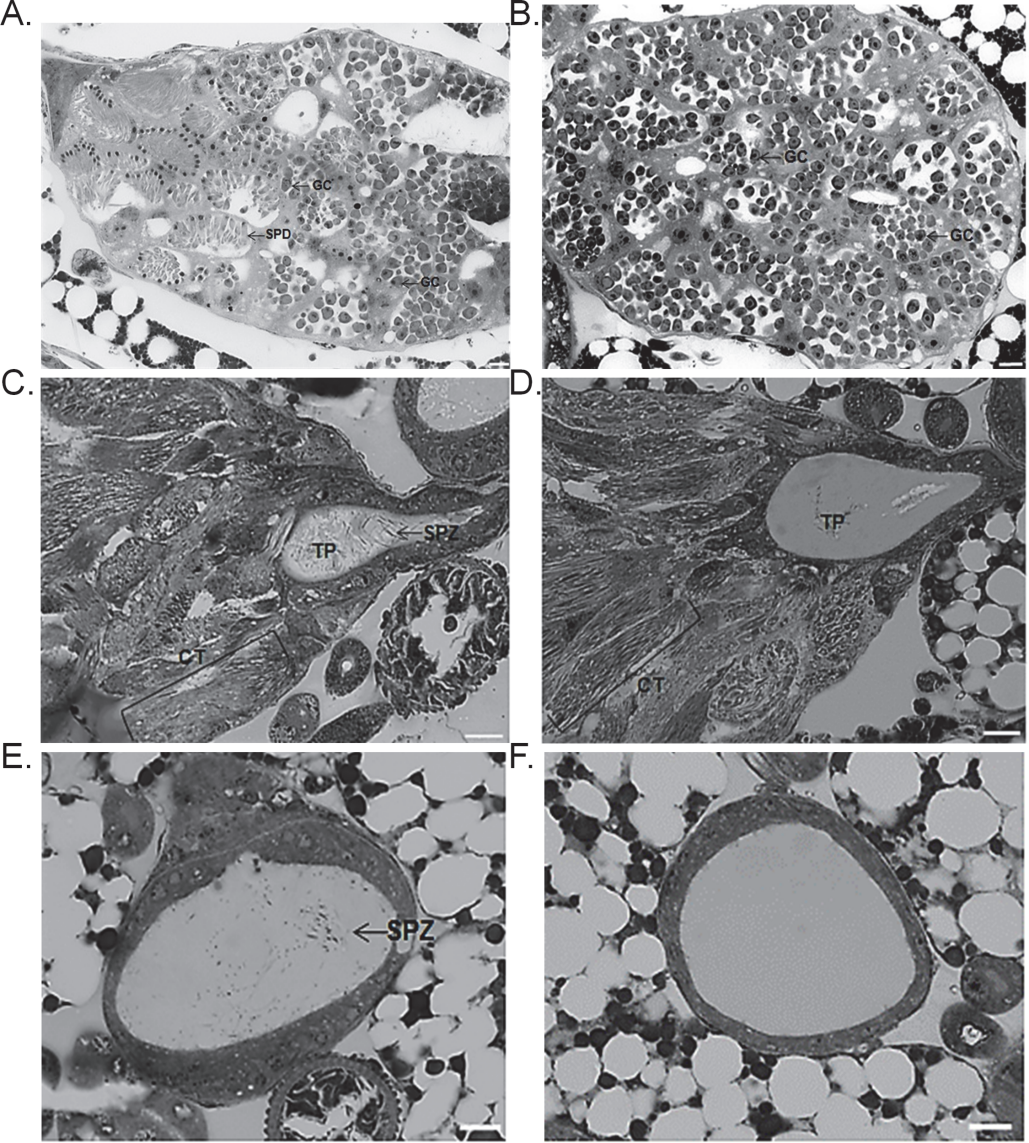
Morphology of the reproductive tract of control and heat-stressed males. A) Morphology of germ cells in cysts from control testes at the second pupal stage. B) Morphology of germ cells in cysts from heat-stressed testes at the second pupal stage (after heat stress). C) Morphology of germ cells in cysts from control testes at the final pupal stage. D) Morphology of germ cells in cysts from heat-stressed testes at the final pupal stage (72 hours after heat stress). D) Morphology of seminal vesicles from control testes at the final pupal stage. E) Morphology of seminal vesicles from heat-stressed testes at the final pupal stage (72 hours after heat stress). GC = Germ Cell, SPD = Spermatid, CT = Cyst, TP = Testis Pocket, SPZ = Spermatozoa. Bar = 10 μm
